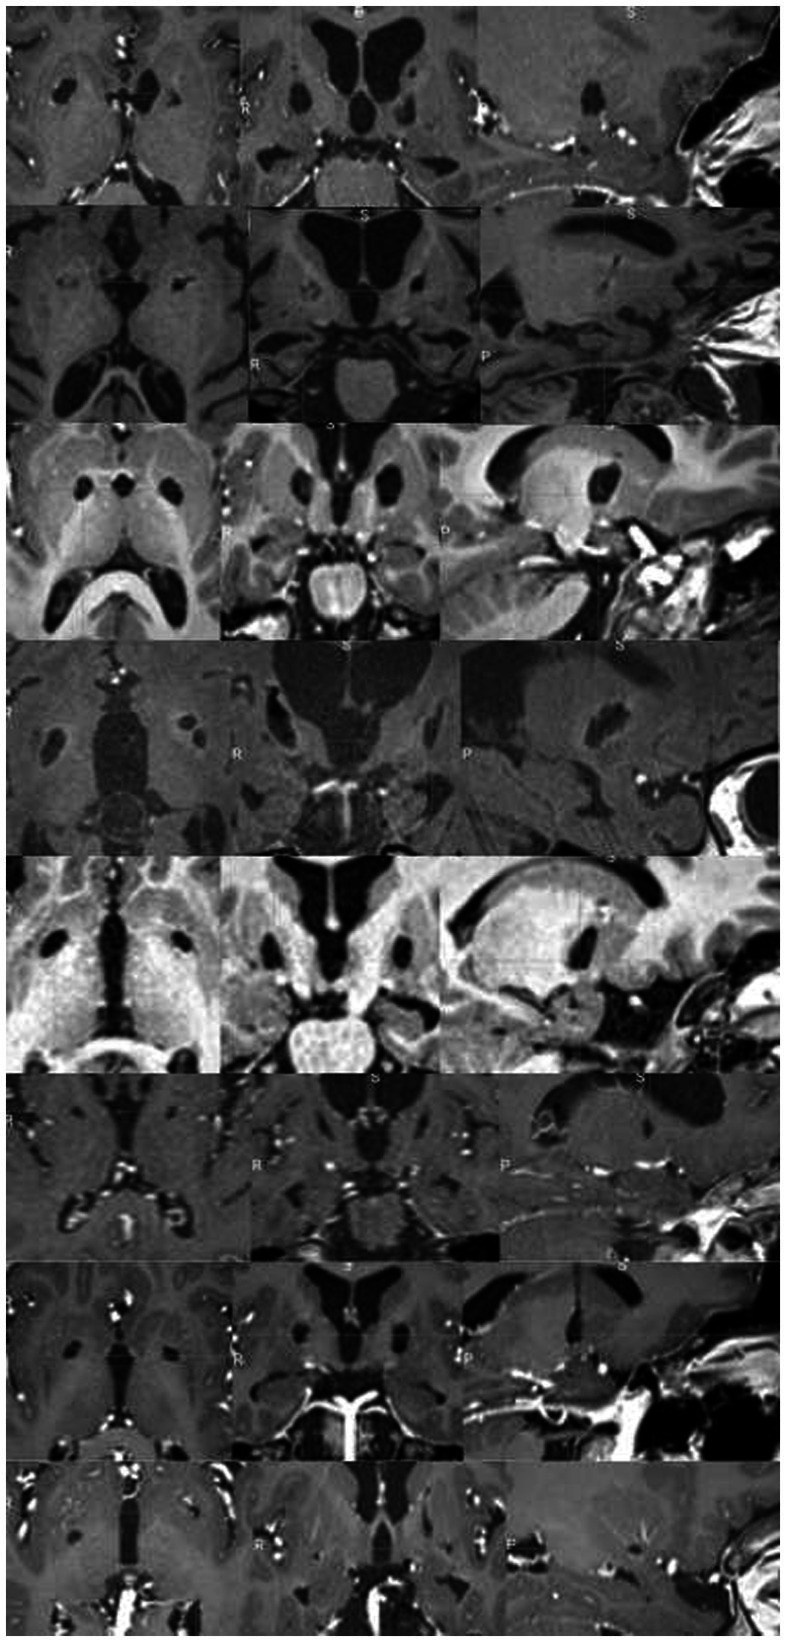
Chronic evolution of GPi lesions in subject undergoing late MRI scans. Each rows represent a single patient T1-weighted MRI sequence on axial, coronal, and sagittal view (from up to bottom: patient #1, #5, #7, #8, #14, #15, #17, #18). In all patients, bilateral lesion was still visible.
